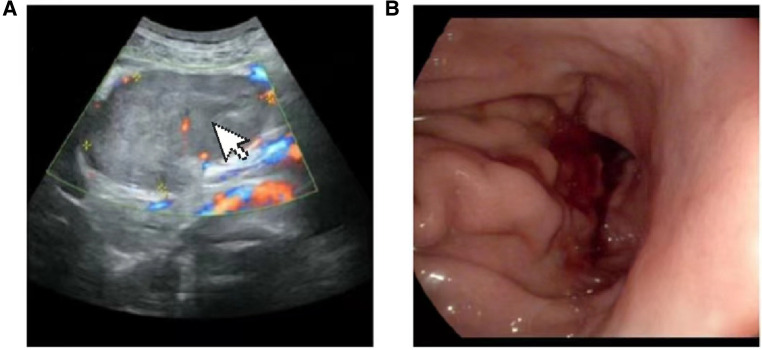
(A) Doppler ultrasound:upper abdominal solid mass. (B) Gastroscopy: chronic atrophic gastritis C1: gastric antrum mass (to be examined).
